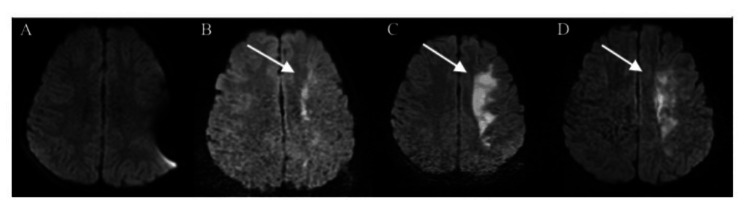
Diffusion-weighted brain magnetic resonance images (MRIs) demonstrating an evolving hyperintensity in the left cerebral hemisphere. Initial MRI from hospital day 0 (A) did not show any signs of ischemia however subsequent MRIs on hospital day 1 (B), hospital day 3 (C) and hospital day 6 (D) demonstrated evolving cytotoxic edema from the ischemic infarct.
